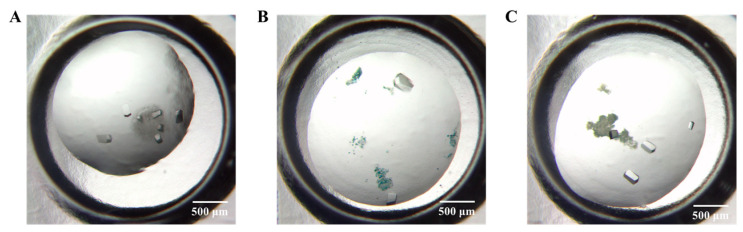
Lysozyme crystallization is facilitated by three types of HKUST-1 coatings at 11 mg/mL lysozyme, 0.1 M sodium acetate (pH 4.25), and 1 M NaCl solution. There are three types of HKUST-1 coatings: water solvent and high pressure (A), alcohol solvent and ultrasound (B), and alcohol solvent and high pressure (C).
